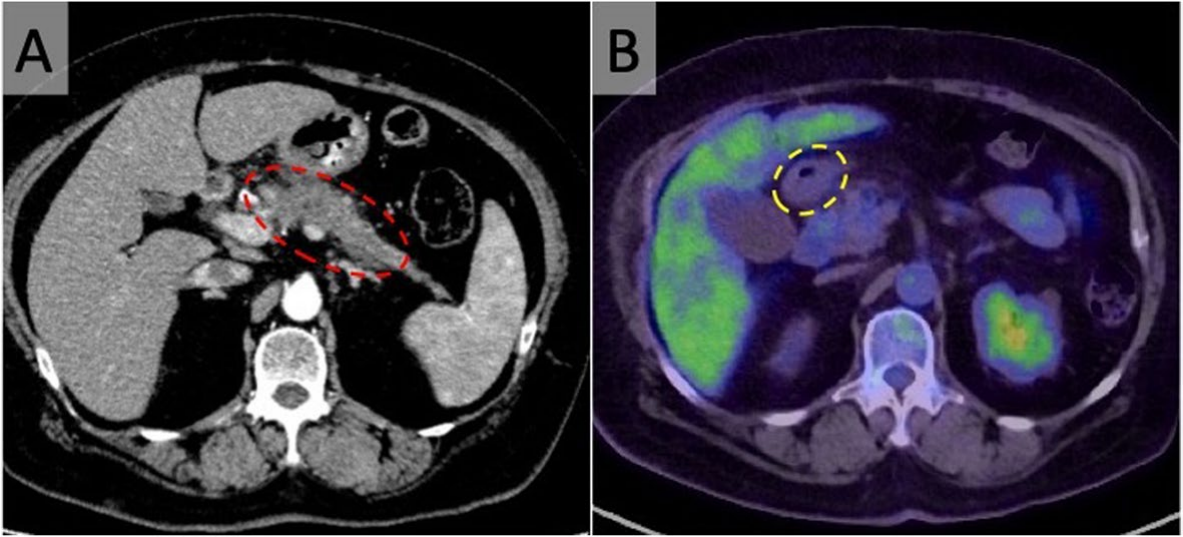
Imaging findings. A Abdominal axial image of computed tomography (CT) shows a tumor with poor contrast in the pancreatic head-body-tail (red dotted line). B Positron emission tomography-CT shows no evidence of distant metastasis, including gastric metastasis. Yellow dotted line indicates the pyloric ring
